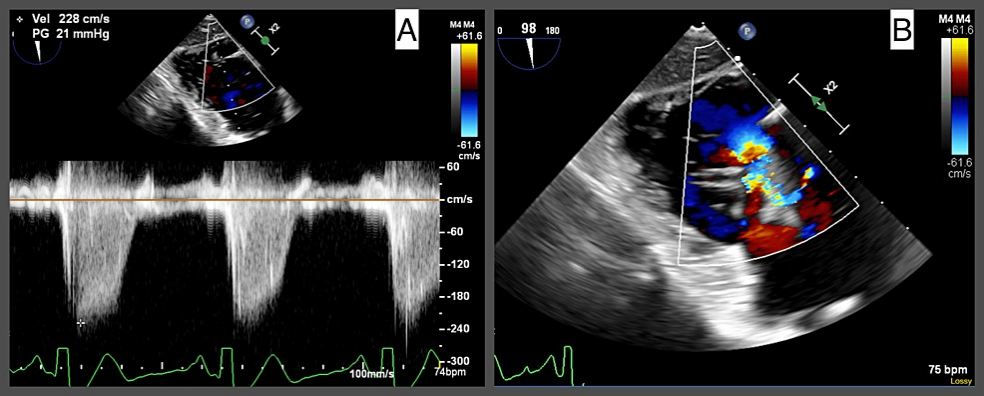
Transesophageal echocardiogram (TEE) evidence of moderate tricuspid regurgitation. Transgastric view. A. Tricuspid regurgitation quantification using continuous wave Doppler velocity. B. Tricuspid regurgitation quantification using color Doppler.
